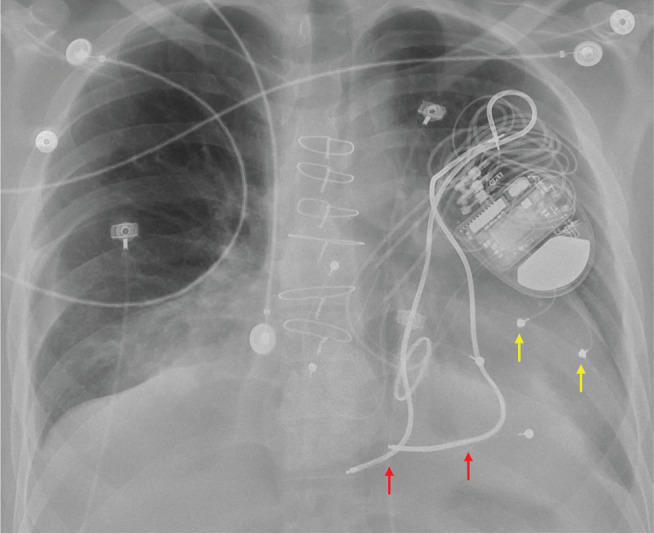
Frontal chest radiograph of a patient with a completely biventricular implantable cardioverter-defibrillator system. Note the pacing leads (yellow arrows) as well as shocking coils (red arrows).
